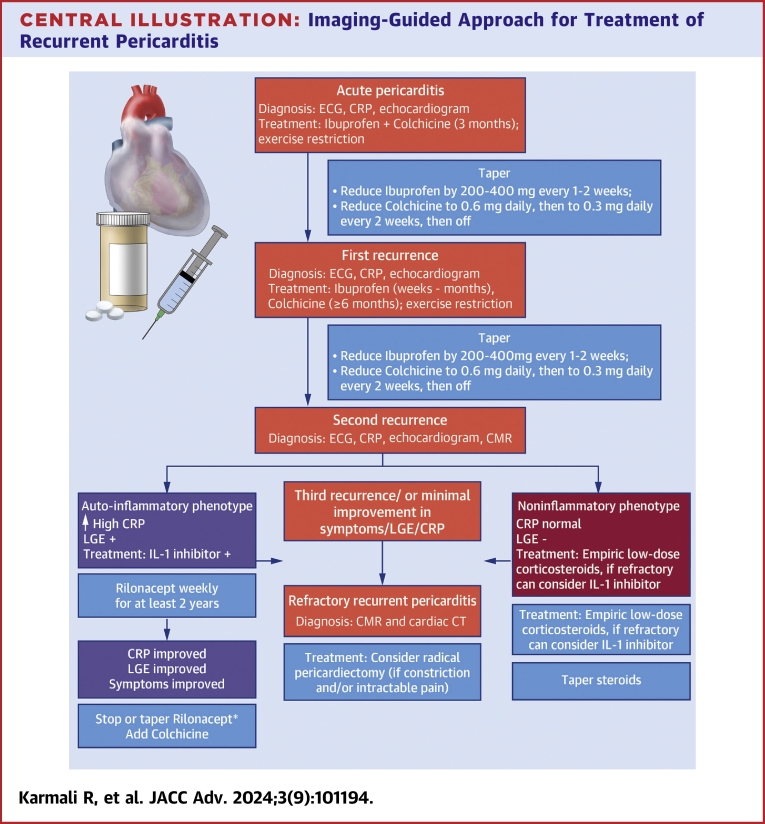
Imaging-Guided Approach for Treatment of Recurrent Pericarditis Following identification of phenotype, a step-wise escalation in approach of therapies is ideal. In the auto-inflammatory phenotype, IL-1 blockers may be considered at an earlier juncture. At our center, ibuprofen is the preferred NSAID and Rilonacept is the preferred IL-1 blocker. ∗Limited data are available regarding stopping or tapering strategy for Rilonacept. Clinicians should engage in shared-decision making with patients on whether to taper the medication gradually or to stop at once following completion of 2 years of treatment. CMR = cardiac magnetic resonance; CRP = C-reactive protein; CT = computed tomography; LGE = late gadolinium enhancement; other abbreviations as in Figures 1 and 3.
